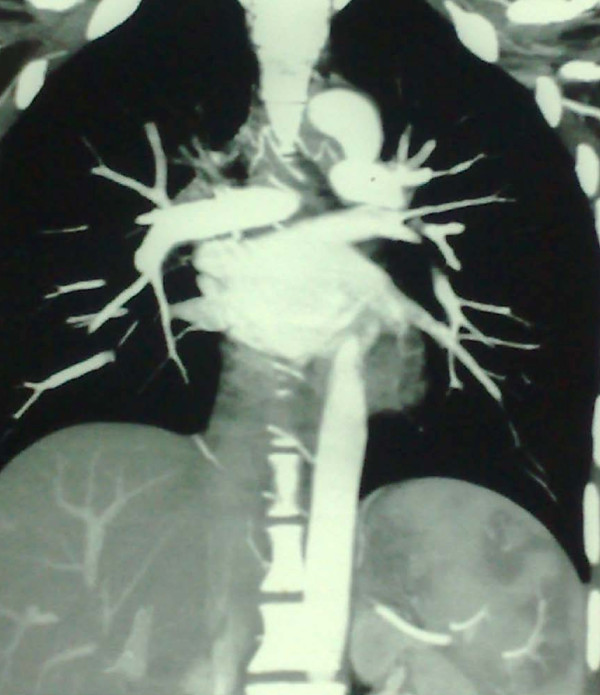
CT-A of arch of the aorta and its major branches showing diffuse intimal thickening, with narrowing of the origins of major branches of the arch of the aorta involving brachiocephalic, right common carotid and left subclavian arteries.
